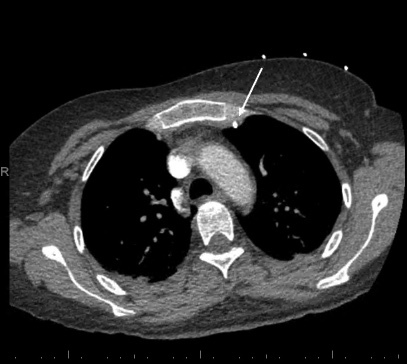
Coronal slice of chest computed tomography demonstrates the central venous catheter within the internal mammary vein, to the left and posterior to the sternum (white arrow).
